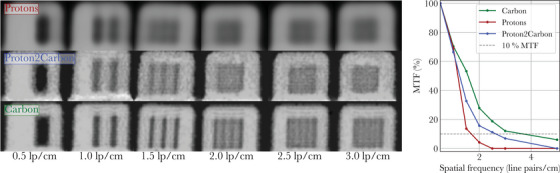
Ion radiographs of line pair modules and associated modulation transfer functions (MTFs). The line pair modules were scanned at the same institution where the training data for the Proton2Carbon model were acquired.
